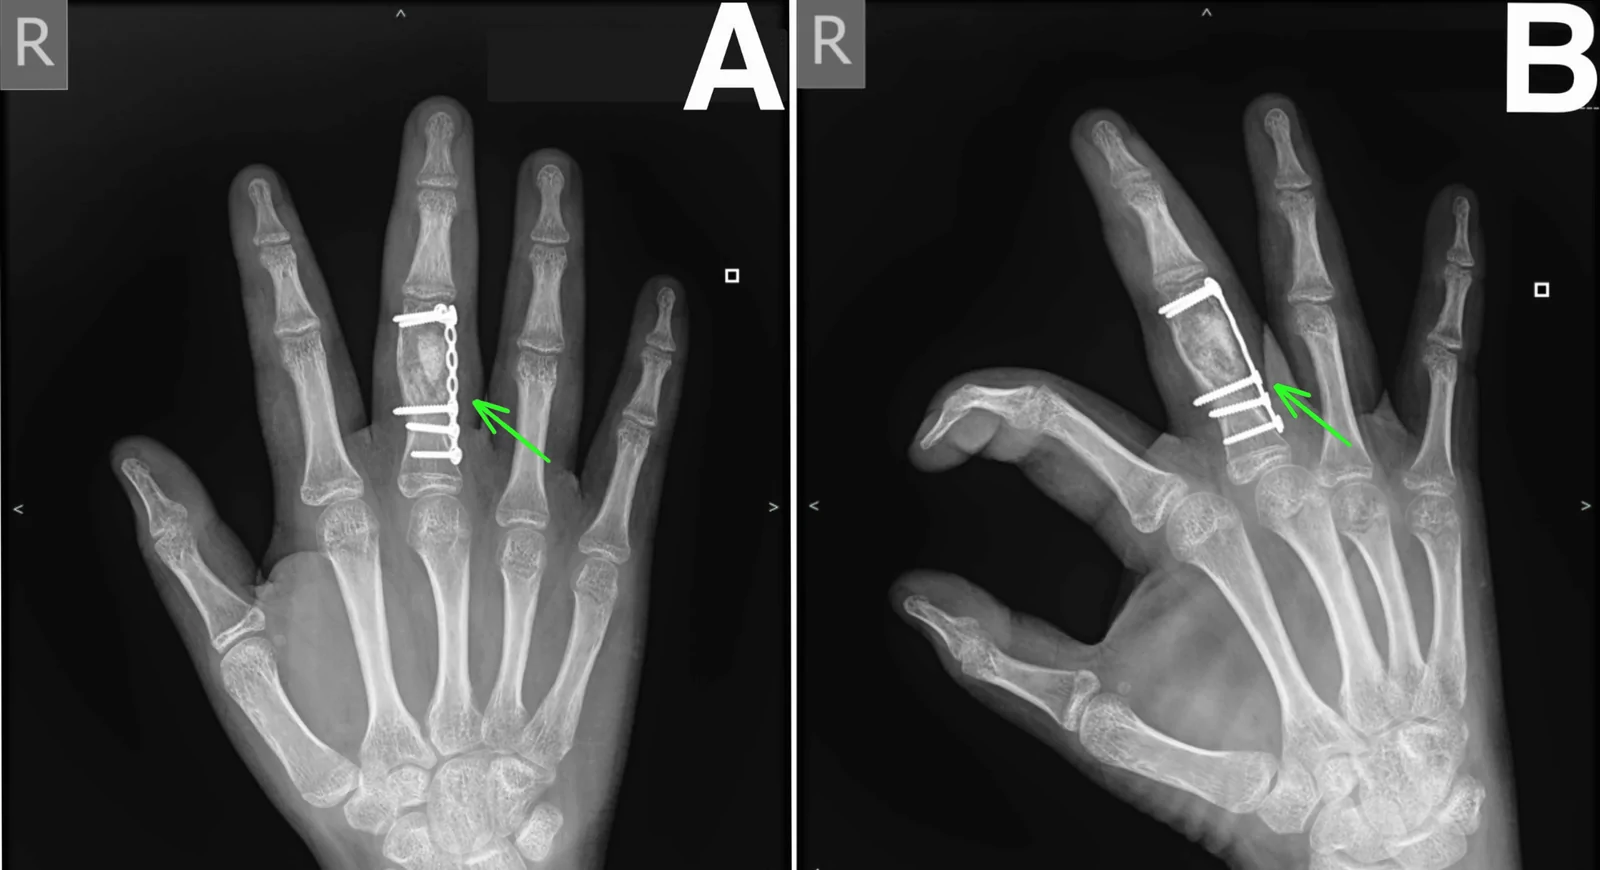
Six-month follow-up radiographs: (A) anteroposterior view; (B) oblique view. Progressive incorporation of the iliac crest autograft with ossification of the bone defect and microfragment plate in situ.
